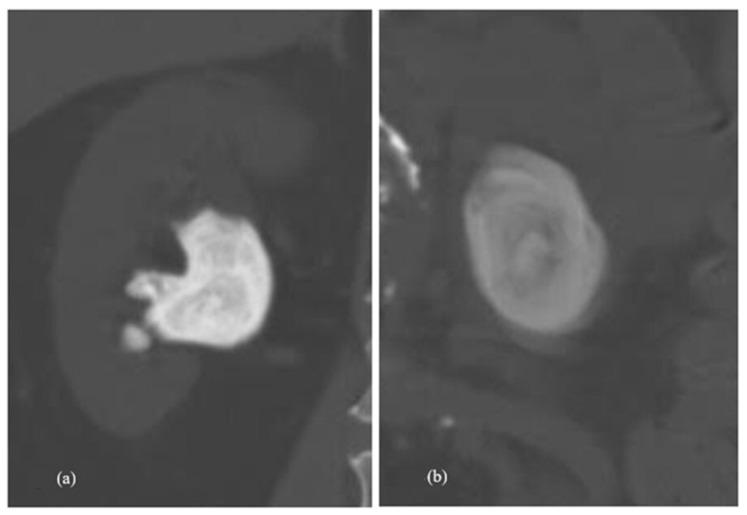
Computed tomography (bone window) of renal calculi. (a) Non-infected renal calculi and (b) infected renal calculi.
